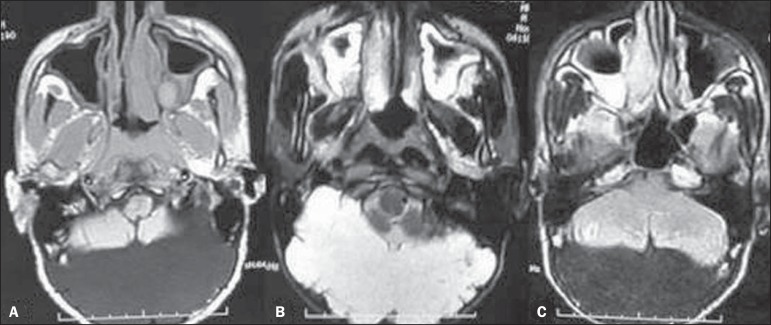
A,B: Axial T1- and T2-weighted MRI scans showing near-total
